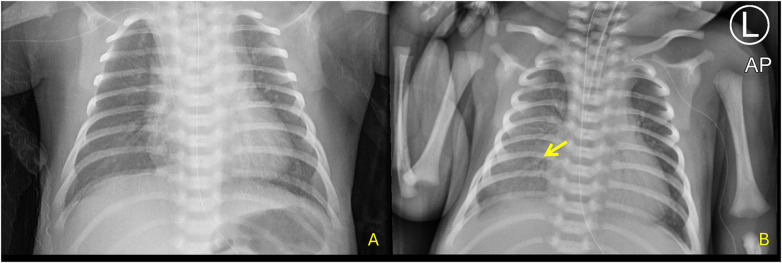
Radiographic progression of the pulmonary sequestration and associated pneumonic changes. (A) Anteroposterior chest radiograph obtained on the first day of life. The image demonstrates bilateral diffuse opacities along the bronchovascular bundles without distinct focal mass lesions or definitive features of pulmonary sequestration. (B) Follow-up anteroposterior chest radiograph on the fifth day of life, showing significant clinical and radiological progression. A large opacity corresponding to the pulmonary sequestration and extensive consolidative changes is visible in the right lung field (indicated by the yellow arrow), with visible air bronchograms.
